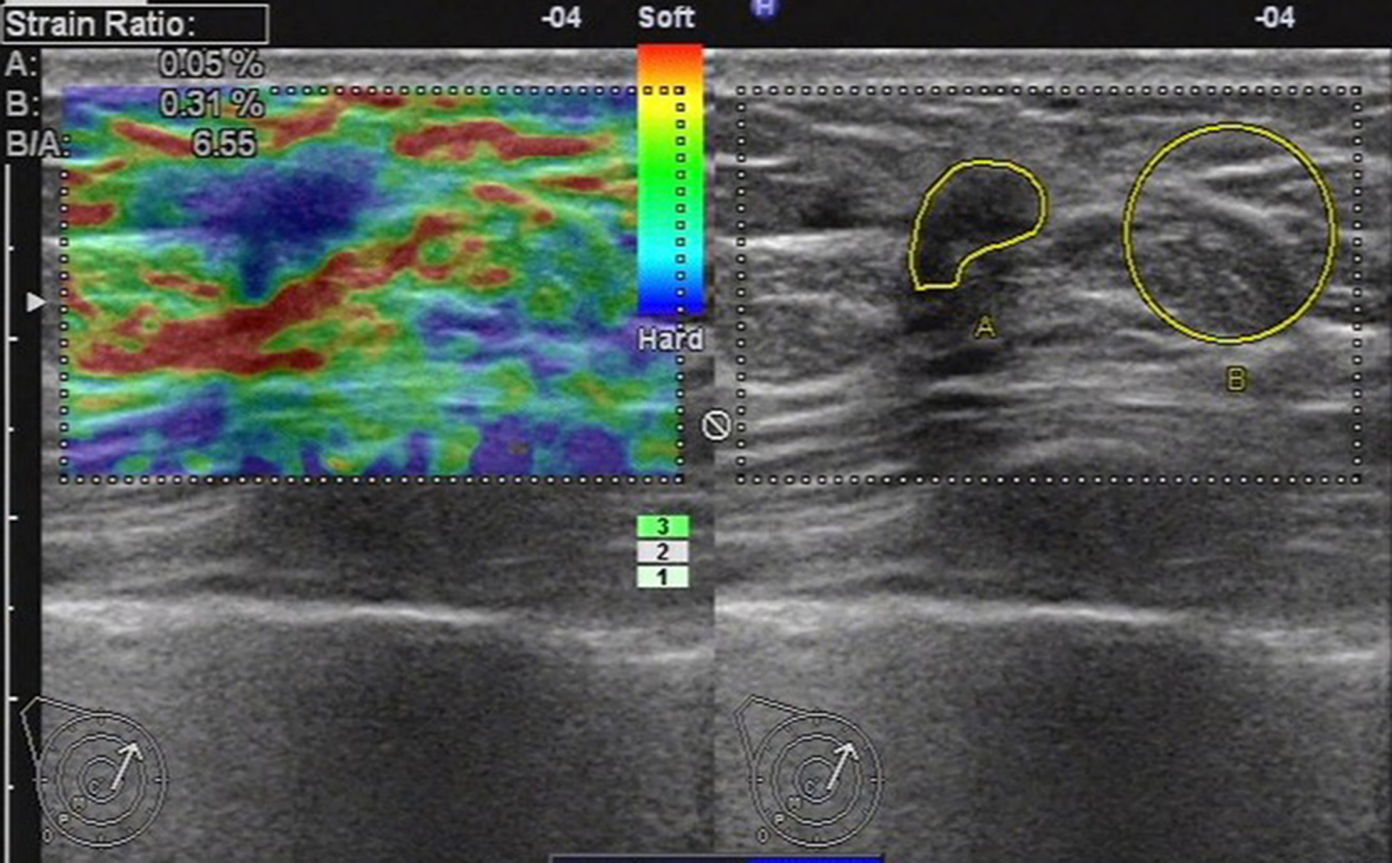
Ultrasonic elastographic image of an IDC in a 47-year-old woman. The lesion was scored 5 with the 5-point scoring method and the maximum diameter of the lesion was 8.2mm. The strain ratio was 6.55 with the strain ratio method at the same breast tissue depth as the reference.
